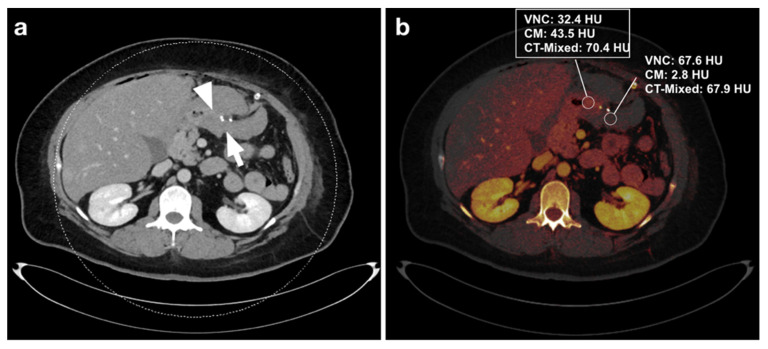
(a) 46-year-old male patient with intra-abdominal hematoma (arrow) adjacent to small bowel loops (arrowhead) after gastric tube surgery. (b) Measurement of iodine maps shows a difference in VNC and iodine values (dual-energy CT) between hematoma and intestinal loops, but no difference in DE-LB (single-energy CT). This case was incorrectly rated as negative in the DE-LB procedure by all 3 readers. After CT, the patient underwent laparoscopy with hematoma evacuation.
